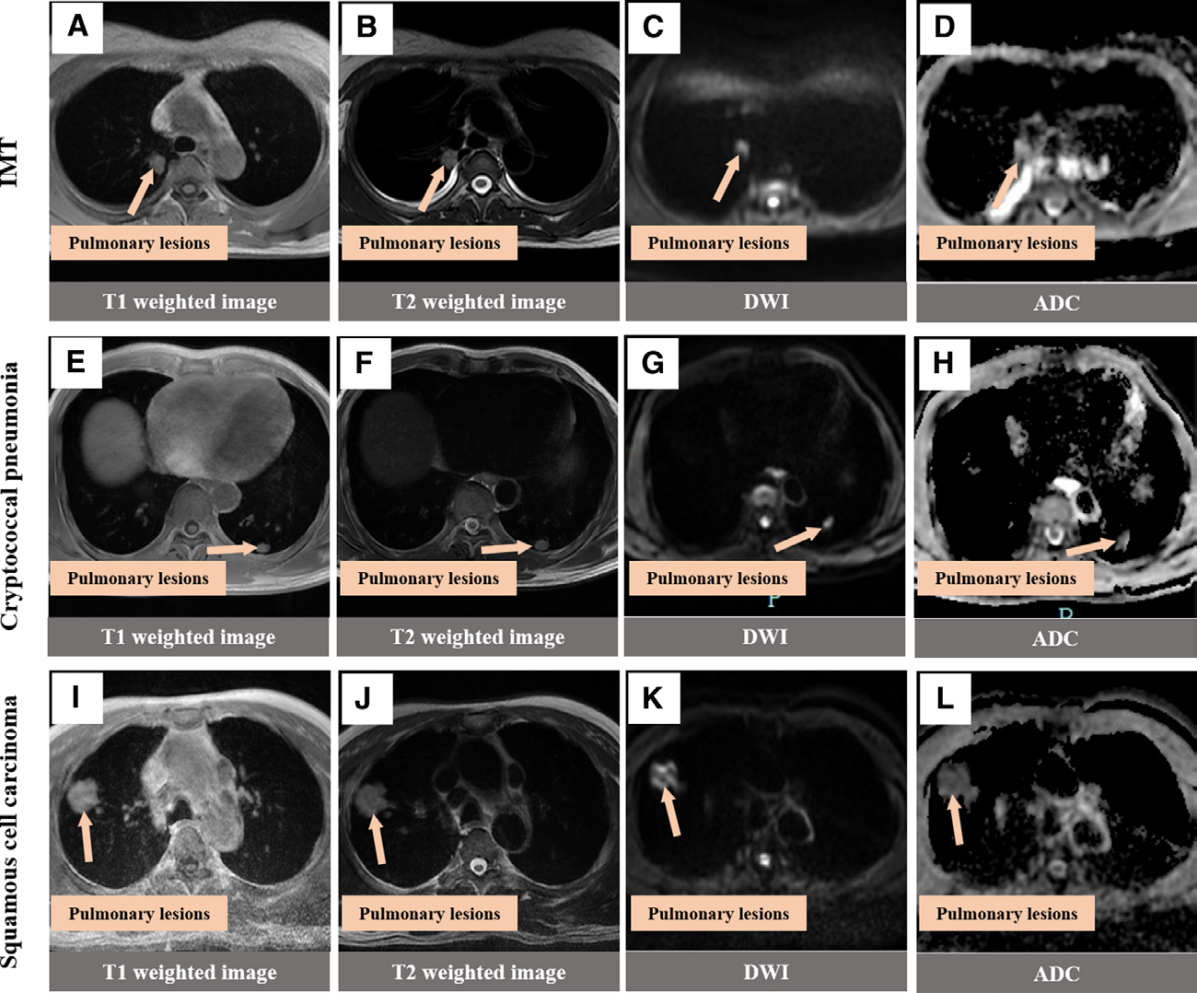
MRI findings of three different types of lung masses Note. (A–D) The T1 and T2 signals of oval shape in the right upper lung were slightly longer, with high signal on DWI and high signal on ADC. (E–H) In the left lower lung, the T1 signal was slightly longer than the T2 signal, the DWI showed a high signal, and the ADC showed a slightly high signal. (I–L) The right upper lung is round, equal to T1 and slightly longer T2 signal, high signal on DWI and low signal on ADC. ADC = apparent diffusion coefficient, DWI = diffusion-weighted imaging, MRI = magnetic resonance imaging.
